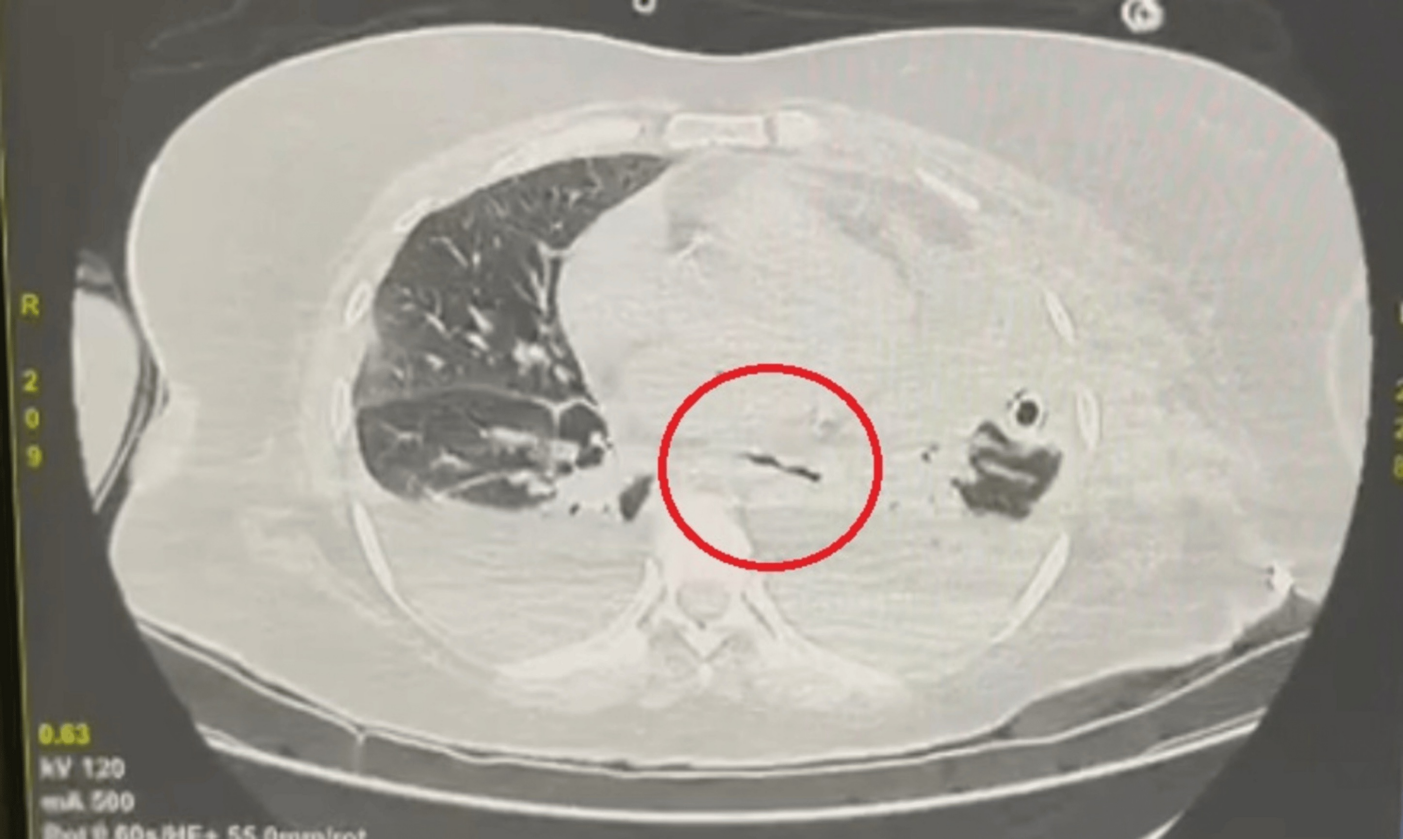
Contrast-enhanced computed tomography of the chest showing the esophageal rupture and leak into the left pleural space and mediastinum
